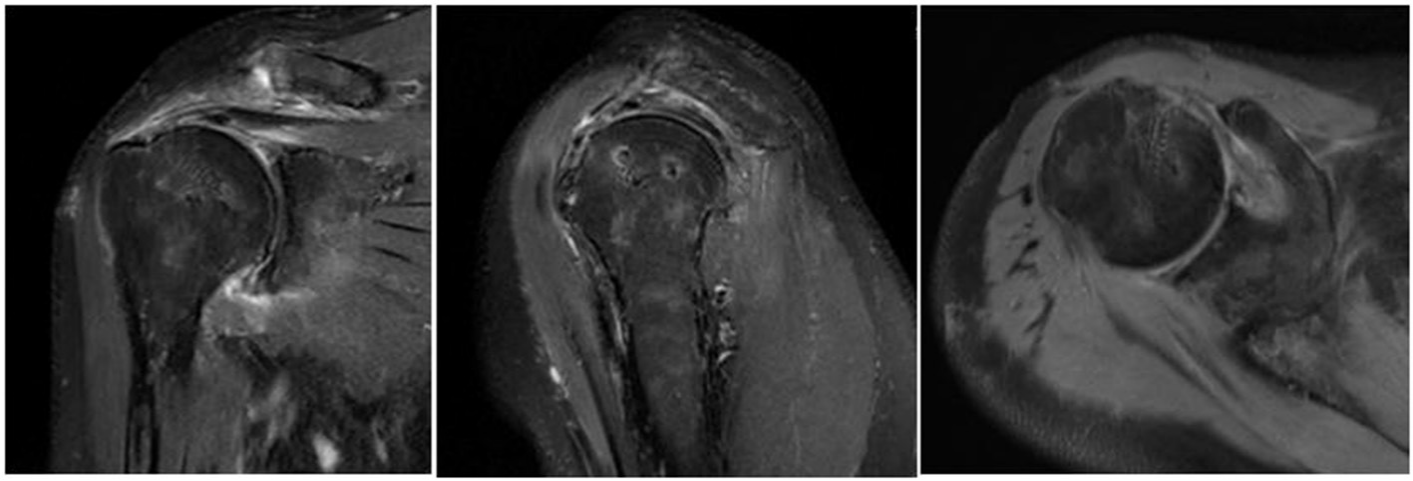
Postoperative MRI images of the control patient.
